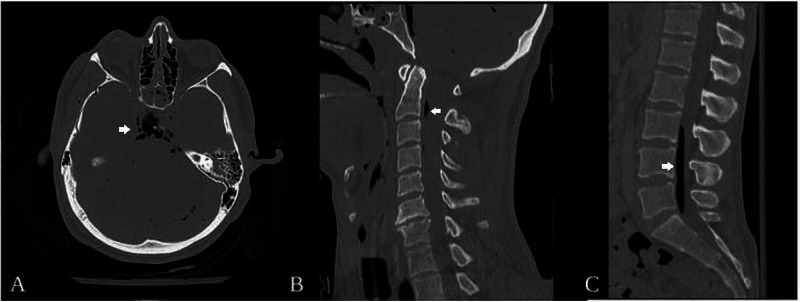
Case 1: Representative Computed Tomography (A) non-contrast head CT axial image demonstrating diffuse pneumocephalus, (B) non-contrast cervical spine CT sagittal reconstruction showing air at the level of C2, and (C) non-contrast lumbar spine CT sagittal reconstruction showing air extending from L3-L5. (CT: computed tomography.)
